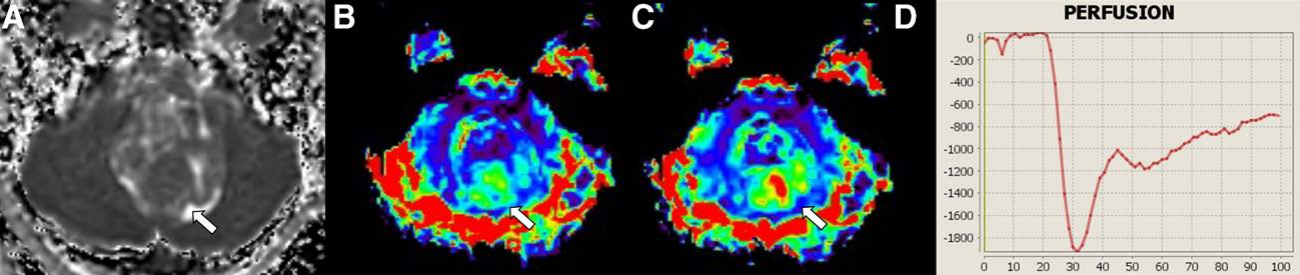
Medulloblastoma in a 21-year-old man. MRI shows a lobulated mass near the fourth ventricle. Normalized mean apparent diffusion coefficient is 1.37 (a, arrow). Normalized relative cerebral blood flow and volume are 1.37 and 2.27, respectively (b and c, arrows). The percentage signal recovery is 62.2 (d). MRI = magnetic resonance imaging.
